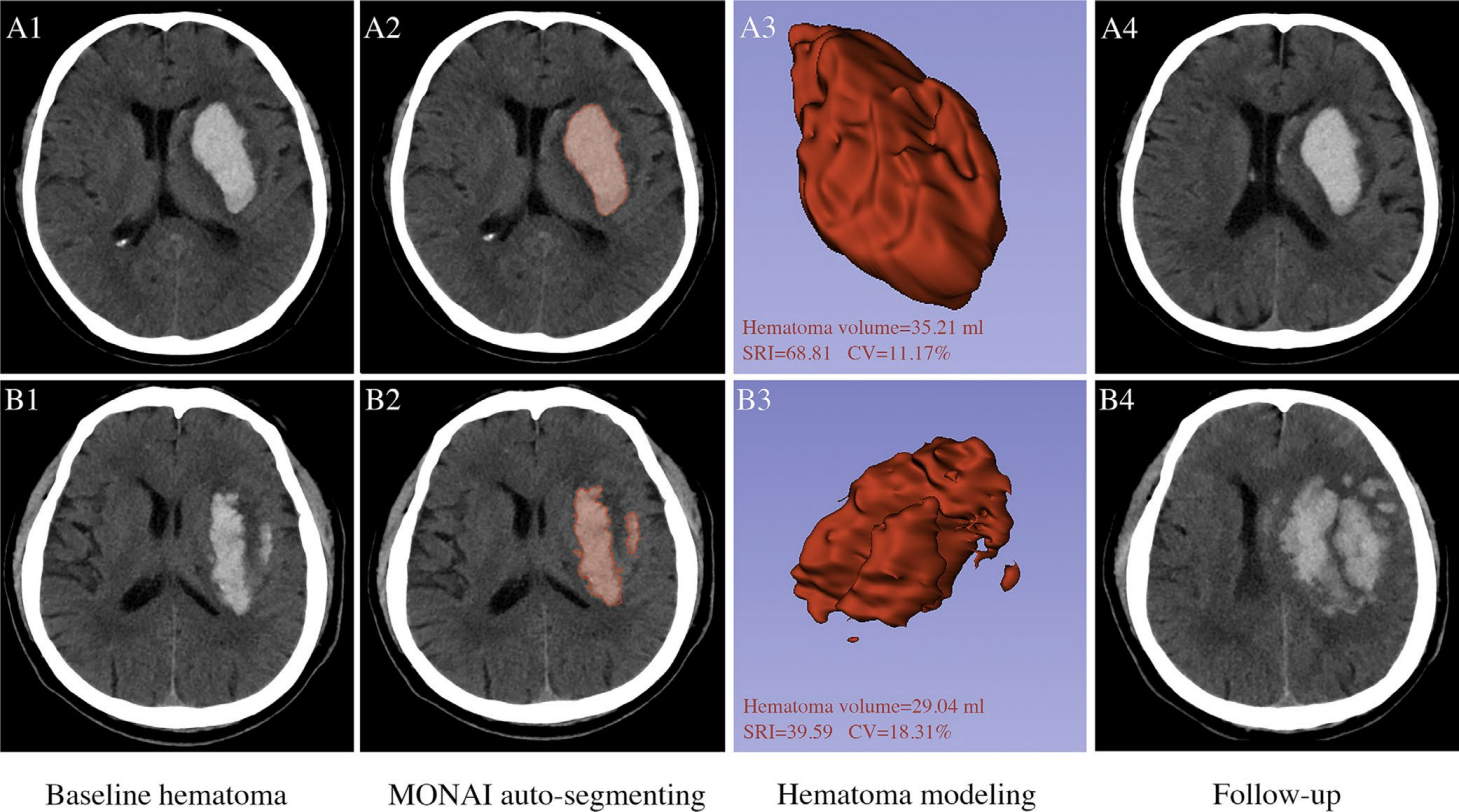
Segmenting and modeling of hematoma utilizing the MONAI label. A1–A4 are images taken from an ICH patient with a high SRI and low DCV of baseline hematoma who did not experience HE. B1–B4 are images taken from an ICH patient with low SRI and high DCV who had HE in follow‐up CT. A1–A3 and B1–B3 show hematoma segmentation and modeling performed using the MONAI label integrating in the 3D‐Slicer software based on baseline CT. The results of the modeled hematoma measurements and calculations (hematoma volume, SRI, and DCV) are presented in A3 and B3. CT, computed tomography; DCV, density coefficient of variation; ICH, intracerebral hemorrhage; SRI, surface regularity index.
